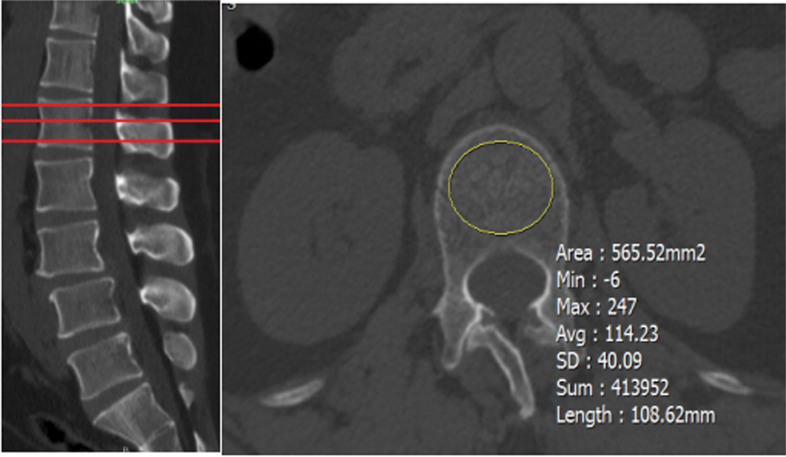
The left panel indicates the selection of the middle L1 vertebral body in the sagittal position of the CT image, and the right panel indicates the placement of the ROI in the mid-level axial position of the L1 vertebral body and the automatic calculation of the mean HU value within the ROI by the PACS system
